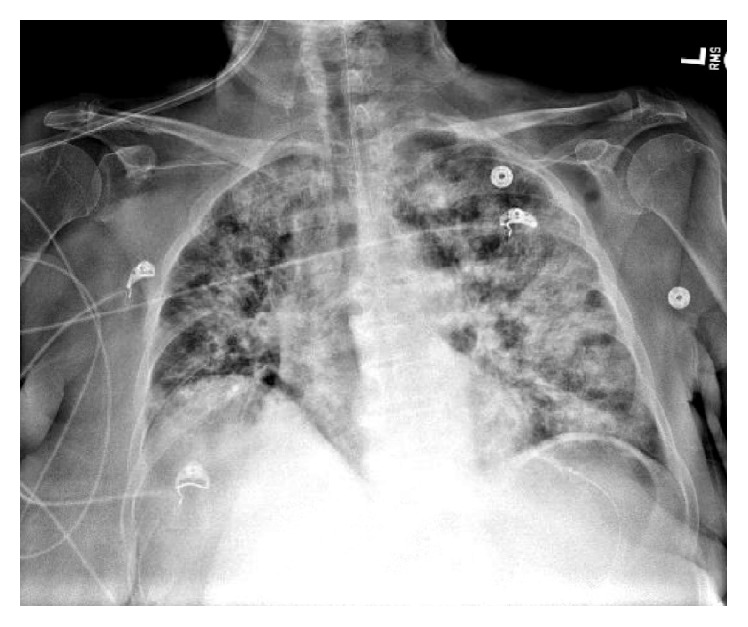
Chest X-ray demonstrating findings suspicious of ARDS.
